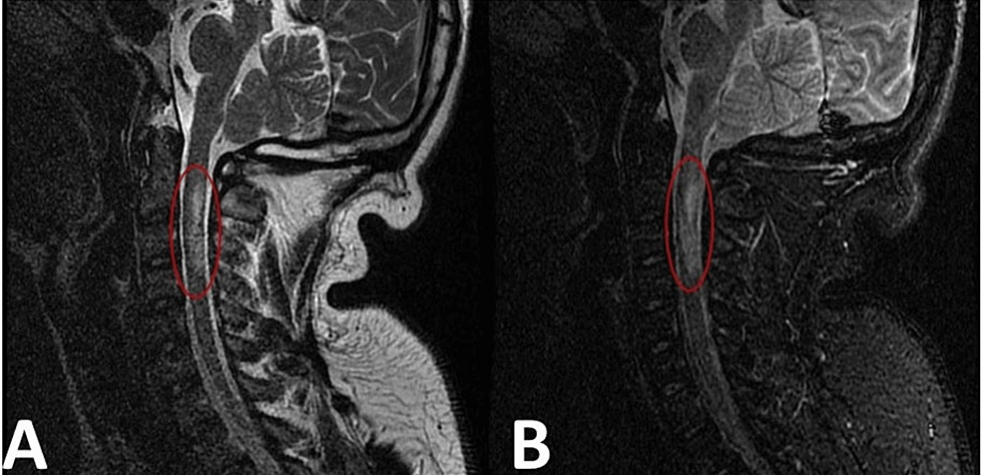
(A) Sagittal T2 MRI of the cervical spine demonstrating a large patchy area of increased T2 signal within the spinal cord at the level of C2-C4 (circled); (B) sagittal T2 STIR MRI of the cervical spine demonstrating a large area of edema within the upper cervical spinal cord from the levels of C2-C4 corresponding to the area of increased T2 signal in (A) (circled). STIR, short tau inversion recovery; MRI, magnetic resonance imaging
